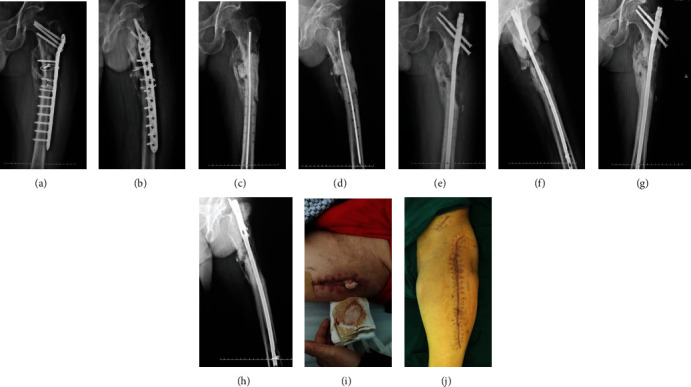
X-ray 1 year after internal fixation of the femoral fracture (a, b), 3 months after Kirschner wire open placement of bone cement (c, d), after the second stage of bone graft internal fixation immediately (e, f), and at 8 months (g, h). General images before (i) and after (j) treatment.
